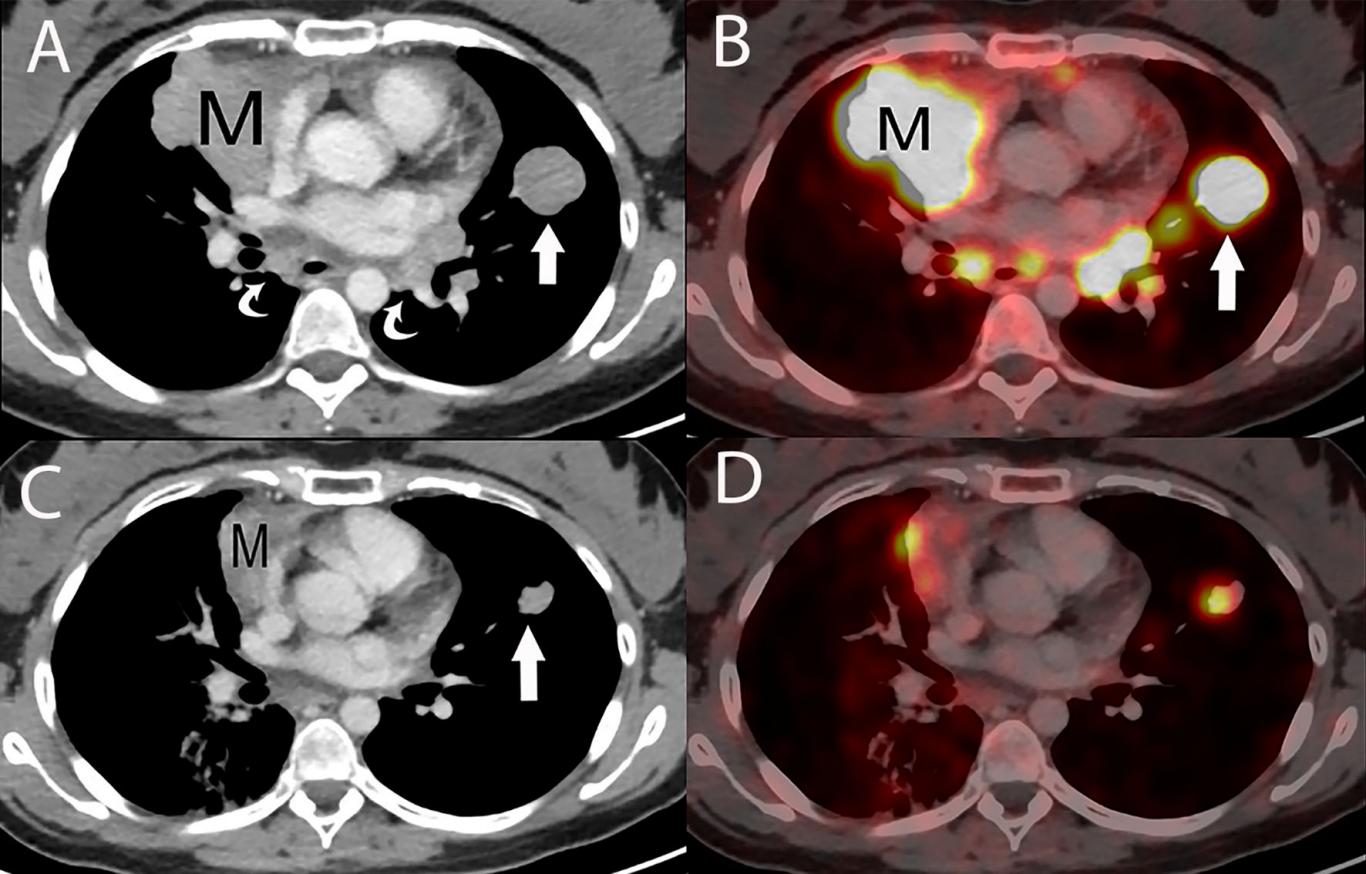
29 year old woman with metastatic thymic carcinoma treated with peptide receptor radiotherapy (PRRT) with ^177^Lutetium. (A) Baseline contrast enhanced chest CT prior to PRRT treatment shows the primary prevascular mass (M), lung metastasis (straight arrow) and metastatic mediastinal lymphadenopathy (curved arrows). (B) Axial fused PET/CT scan at the same level as A, using a somatostatin analogue, Ga ^68^-DOTATATE, reveals DOTATATE uptake in the primary mass (M), lung metastasis (straight arrow) and mediastinal lymphadenopathy. (C) Contrast enhanced chest CT, following two peptide receptor radiotherapy (PRRT) with ^177^Lutetium sessions show an impressive partial response with a decrease in size of the primary mass (M) and of the lung metastasis (straight arrow), with resolution of the mediastinal lymphadenopathy. (D) Axial fused Ga ^68^-DOTATATE PET/CT scan at the same level as C, shows a corresponding marked decrease in DOTATATE uptake consistent with an impressive metabolic partial response.
